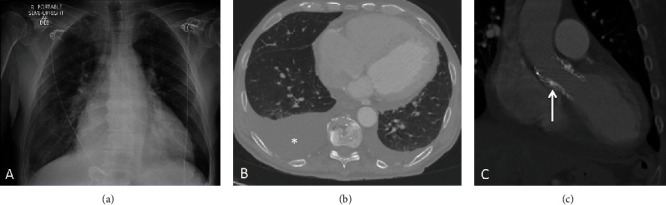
Radiographic studies. (a) Chest X-ray with prominent cephalization of the pulmonary vasculature in favor of pulmonary edema. CT scan showing (b) a moderate right side pleural effusion (asterisk) and (c) low-lying percutaneous aortic valve (arrow).
